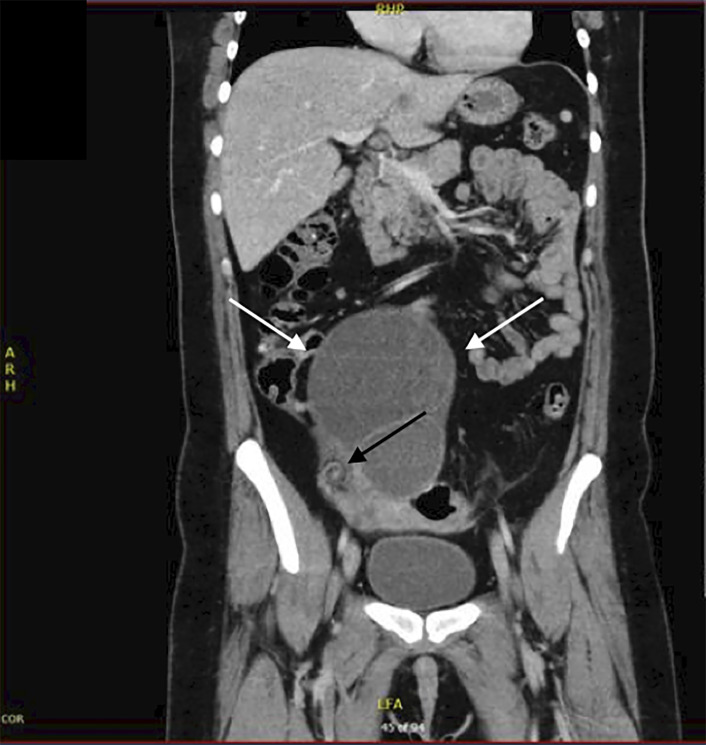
Coronal view of contrast-enhanced computed tomography in a woman with four days of right lower quadrant/pelvic pain, showing the “whirlpool sign” of ovarian torsion (black arrow), confirmed at laparoscopy. Also shown is 10 × 15 centimeter (cm) right ovarian cystic mass (white arrows).
